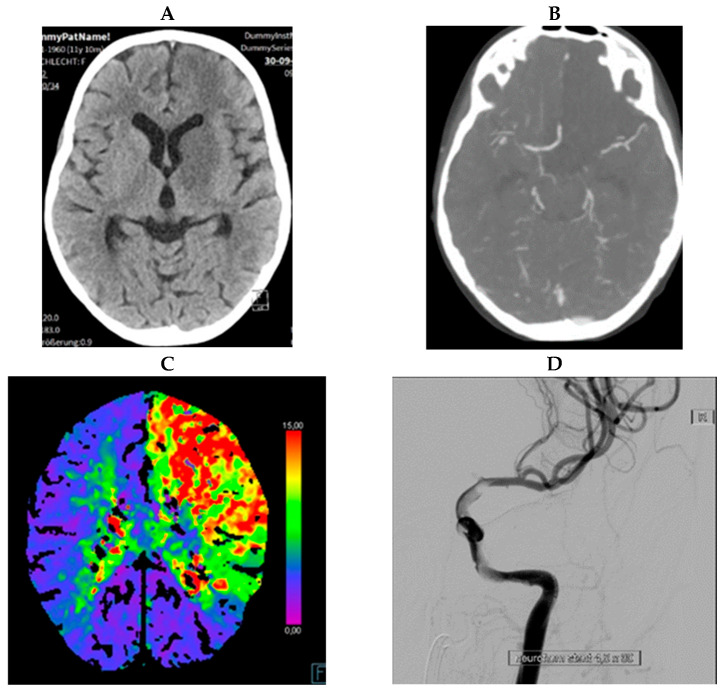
Computed tomography (CT) and magnetic resonance (MR) imaging on day +1. (A), axial CT imaging showing infarction in the perfusion area of the left anterior (ACA) and middle cerebral arteries (MCA). (B), CT angiography showing the extension of the occlusion of left internal carotid artery into the proximal left anterior and middle cerebral arteries. (C), time-to-drain (TTD) documents extensive perfusion deficits in the left frontotemporal area. (D), postprocedural angiography following interventional thrombectomy and stenting of the left internal carotid artery.
